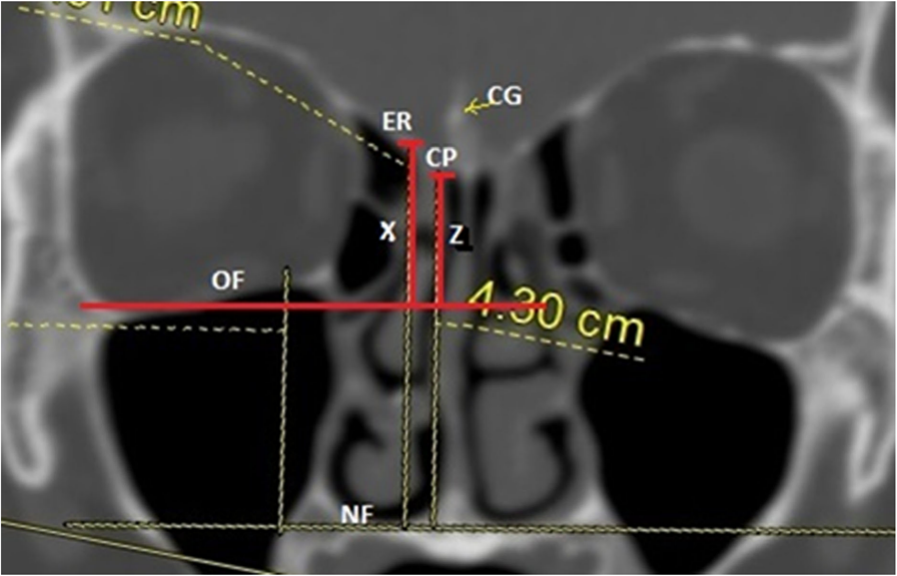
CT scan of paranasal sinus (coronal view) showing the relation of orbital floor from the other anatomical landmarks. (CG- crista galli; ER- ethmoid roof; CP- cribriform plate; OF- orbital floor; NF- nasal floor; X-ethmoid roof to orbital floor; Z- cribriform plate to orbital floor)
